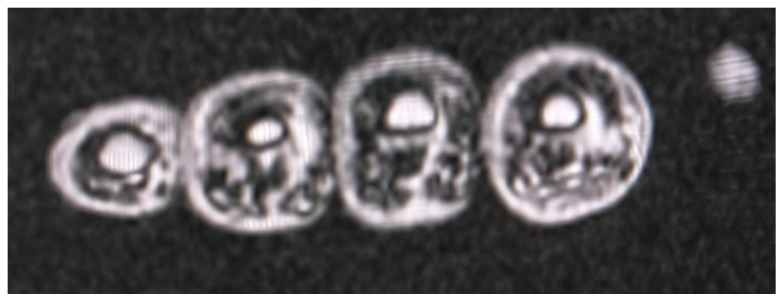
MRI of the hand—hypointense cutaneous thickening on T1, no synovitis or joint effusion.
